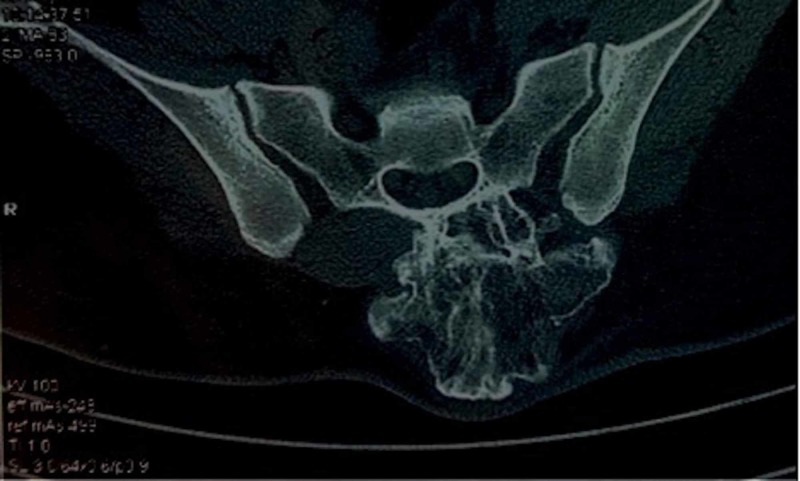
Transverse cut section of the CT scan shows a cauliflower-like bony outgrowth arising from the posterior aspect of the sacrum on the left side
